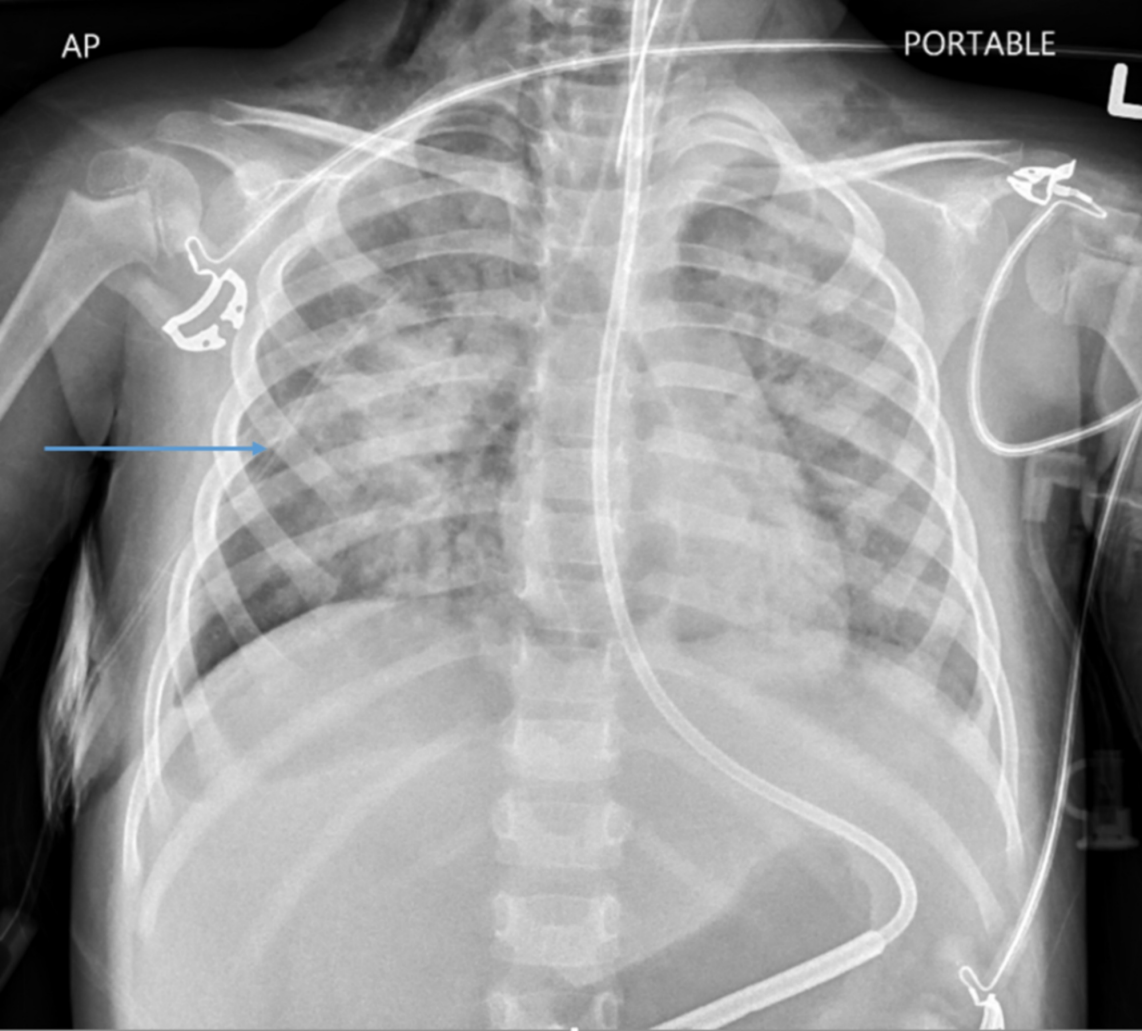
Chest X-ray showing the thoracostomy tube.
